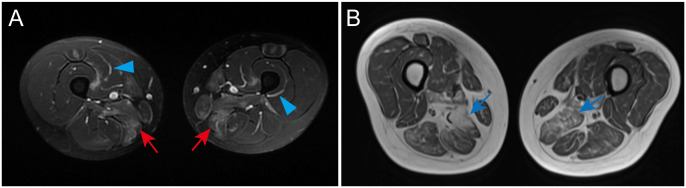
Examples of a thigh and lower leg MRI in SRP-IMNM. (A), a T2-weighted image shows obvious edema in the adductor magnus, gracilis, and sartorius of the thigh (red arrow) and fascial edema (blue arrowhead). (B), a T1-weighted image reveals evident muscle atrophy and fatty replacement in the posterior muscles of the thigh (blue arrow). These pictures are cited from our previous study (7).
